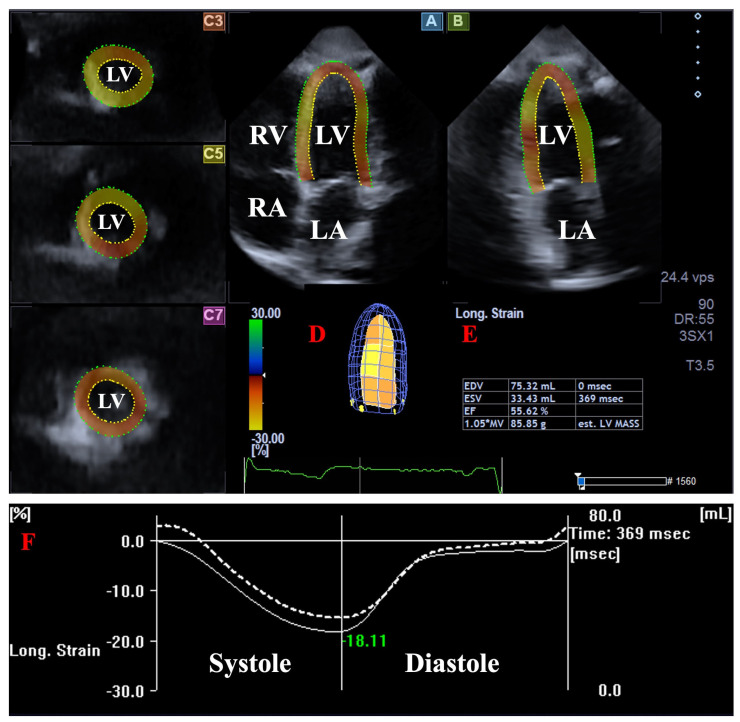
Assessment of left ventricular (LV) volumes using three-dimensional (3D) speckle-tracking echocardiography. The apical longitudinal four-chamber (A) and two-chamber (B) views, along with short-axis views at the apical (C3), mid-ventricular (C5), and basal (C7) LV levels, are displayed alongside a virtual 3D LV cast (D) and the corresponding calculated volumetric data (E). Time–LV volume change curve (dashed white curve) together with time–LV longitudinal strain curve (white curve, not analysed in this study) are presented in (F). Abbreviations: LA = left atrium; LV = left ventricle; RA = right atrium; RV = right ventricle; EDV = end-diastolic volume; ESV = end-systolic volume; EF = ejection fraction.
